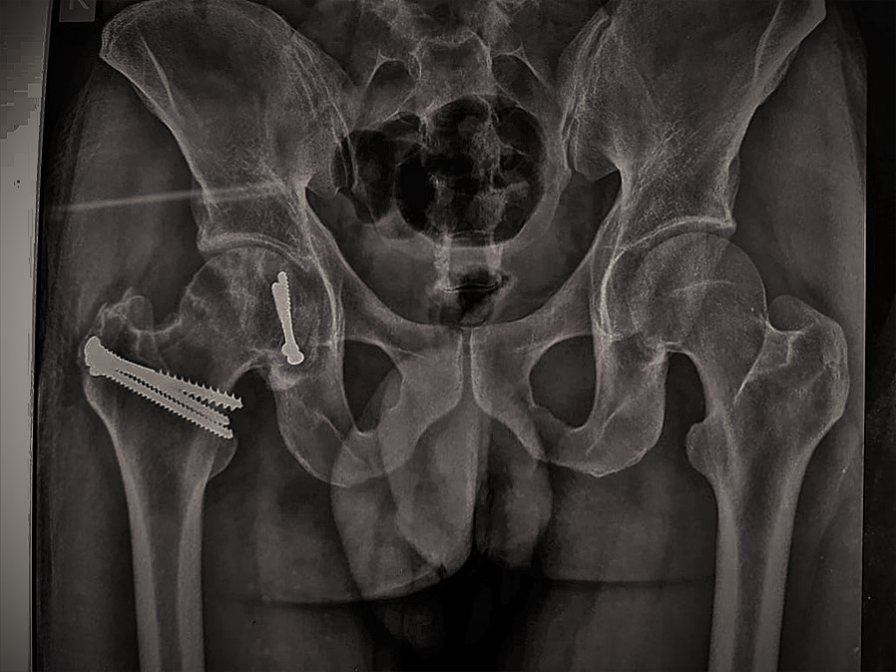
Follow-up radiograph at 12 months showing femoral head osteonecrosis
